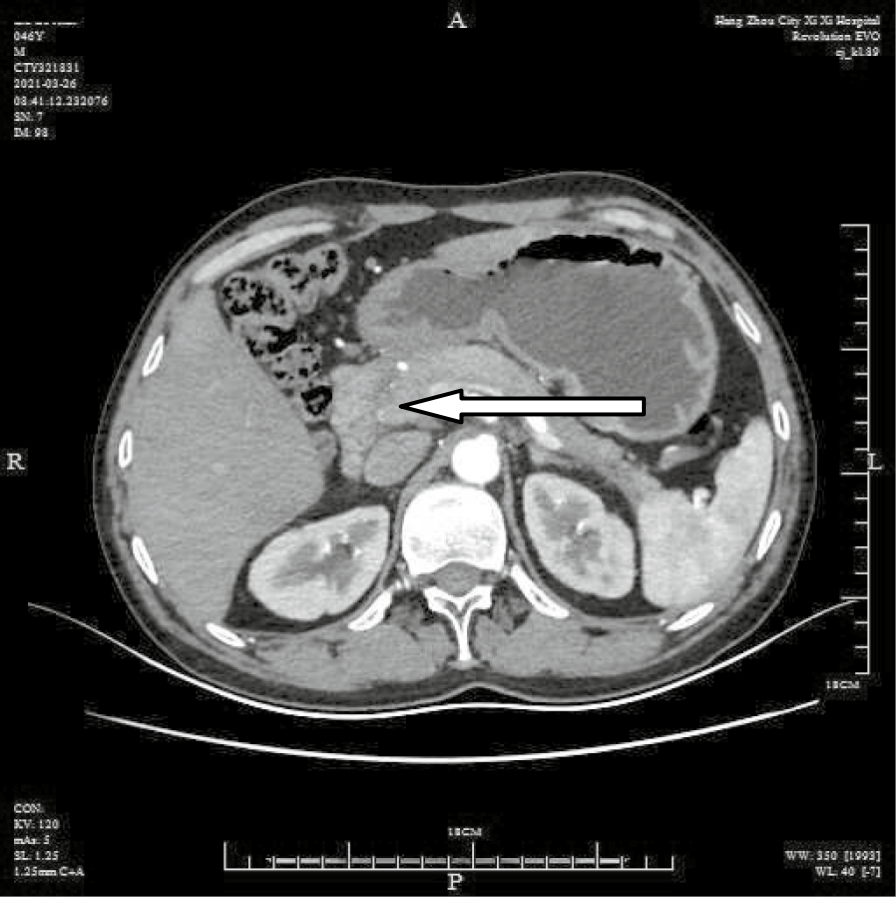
Head pancreas normal in CT after one month treatment
